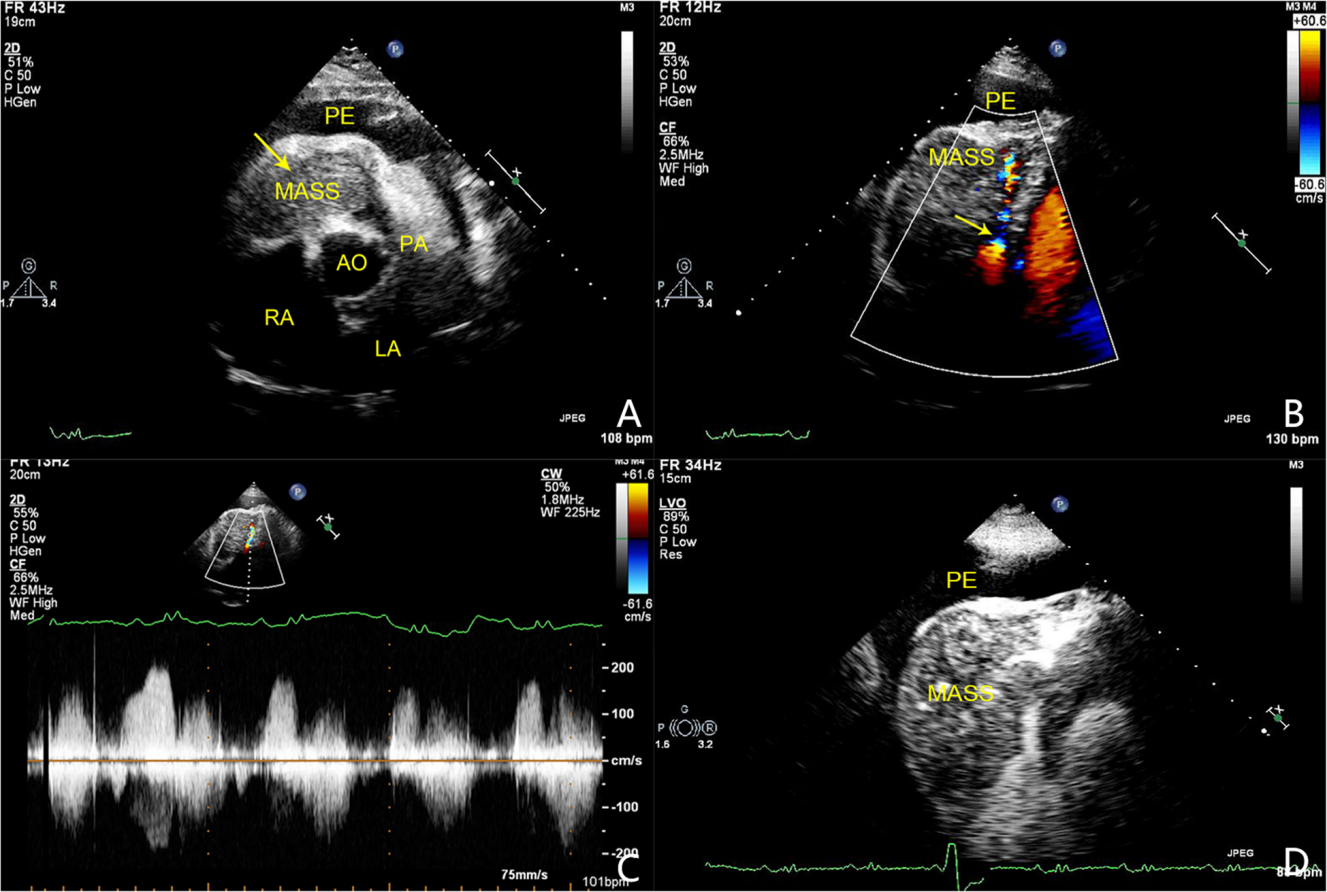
Echocardiographic findings. A huge mass occupying almost the whole RVOT (yellow arrow). Massive pericardial effusion can be observed. (A); Color Doppler showed fine and high velocity flow in cavity and inflow tract of RV. (B); Continuous-wave Doppler spectrum of the high-velocity flow in the inflow tract of RV, with the peak velocity of 2 m/s.(C); CE showed higher enhancement of contrast agent in the mass than the myocardium.(D). (AO: aortic artery, CE: contrast echocardiography, LA: left atrium, PA: pulmonary artery, PE:pericardial effusion, RA: right atrium)
